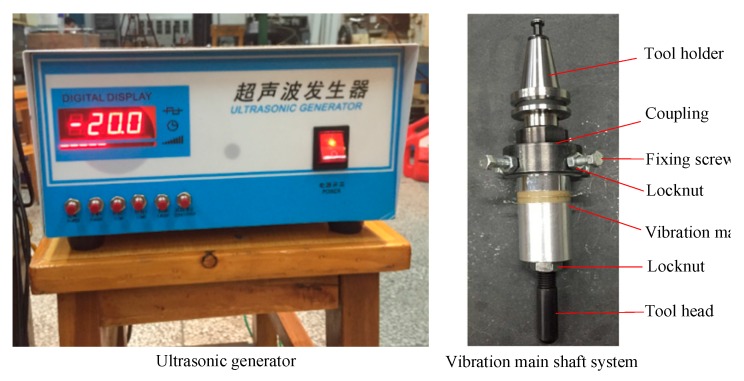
Ultrasonic vibration system.
